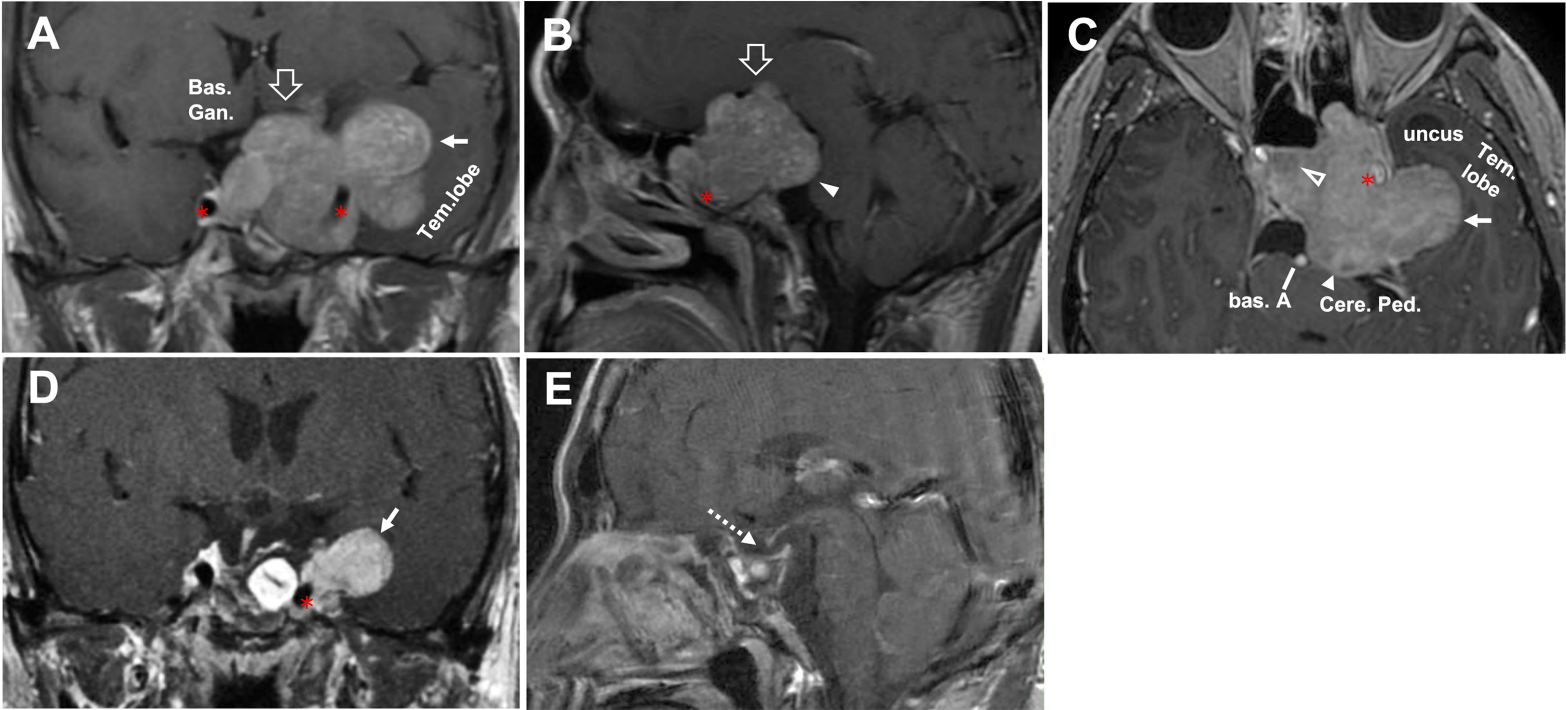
CASE4, The superior-posterior-lateral compartment extension. A 45-year-old man with a giant unfunctional pituitary adenoma. CC has severe visual field defects, ophthalmoplegia, hypopituitarism, and severe headache. (A–C), Preoperative coronal (A), sagittal (B), and axial (C) T1-weighted contrast-enhanced MRI showing a pituitary adenoma with left-side cavernous sinus invasion (Knosp grade 4) and extension through the superior (open arrow, in A, B), lateral (solid arrow, in A, C), and posterior (solid arrowhead, in B, C) compartments. The disorderly extension of the tumor had caused compression in basal ganglia(A) by the superior aspect of the tumor. It compressed the midbrain (cerebral peduncle) by the posterior aspect (B, C). It caused a significant displacement of the temporal lobe (A, C). The tumor’s relationship with ICA (asterisk) was given, and tumor (A, C) encased the left side of the ICA (asterisk). After removing the intrasellar aspect (open arrowhead, in C) of the tumor, we deal with the rest part following an arrangement decided by the surgeon’s preference as “superior—posterior—lateral.” (D–E), Postoperative T1-weighted contrast-enhanced MR images showing residual tumor of lateral aspect (solid arrow, in D) and total resection for the rest aspect (dotted arrow, in E). A planned secondary-stage transcranial surgery was performed to resect the residual tumor lateral portion. ICA, internal carotid artery; bas. A, basilar artery; bas.gang., basal ganglia; cere. ped., cerebral peduncle; tem., temporal.
